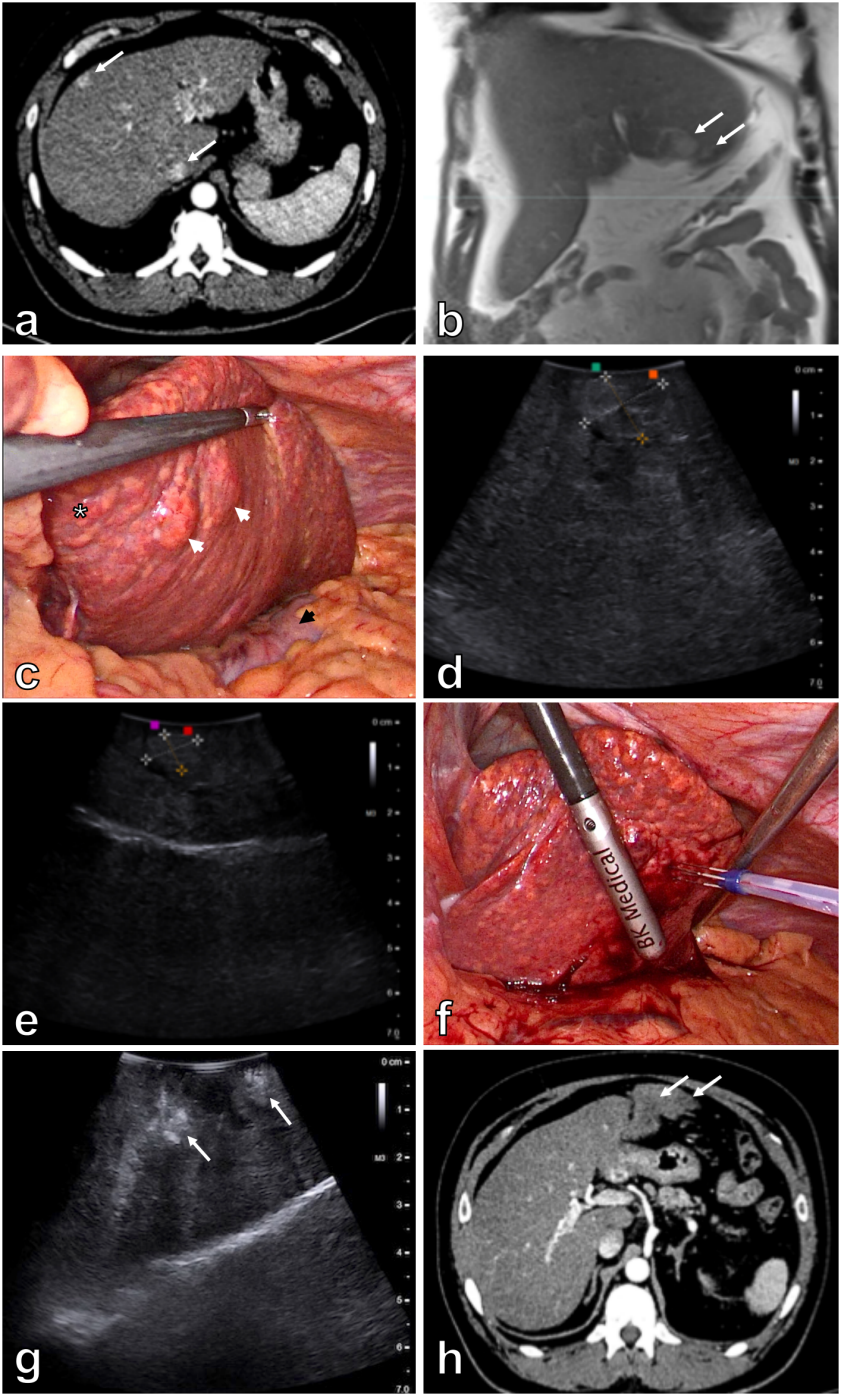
Patient 2. Initial contrast enhanced abdominal CT demonstrates multiple hypervascular lesions (white arrows) in the cirrhotic liver (A). Contrast enhanced MRI of the liver after 24 cycles of immunotherapy demonstrates 18 mm and 8 mm lesions (white arrows) in segment III of the liver (B). Laparoscopy shows macronodular liver cirrhosis (asterisk). Both tumors (white arrowheads) are seen after the retraction of the left liver lobe. Notice the stomach just underneath the left liver lobe (black arrowhead) (C). Intraoperative US better demonstrates a larger 20 x 15 mm tumor (D) and a smaller 8 x 12 mm tumor in the segment III (E). Electric pulses to the tumor are applied through convergent Stinger electrode. Placement of the electrode is aided by intraoperative US (F). Control intraoperative US immediately after the treatment demonstrates areas of avital lesions (white arrows) (G). Contrast enhanced abdominal CT three months after the procedure demonstrates 22 mm and 10 mm avascular lesions (white arrows) in segment III of the liver (H).
